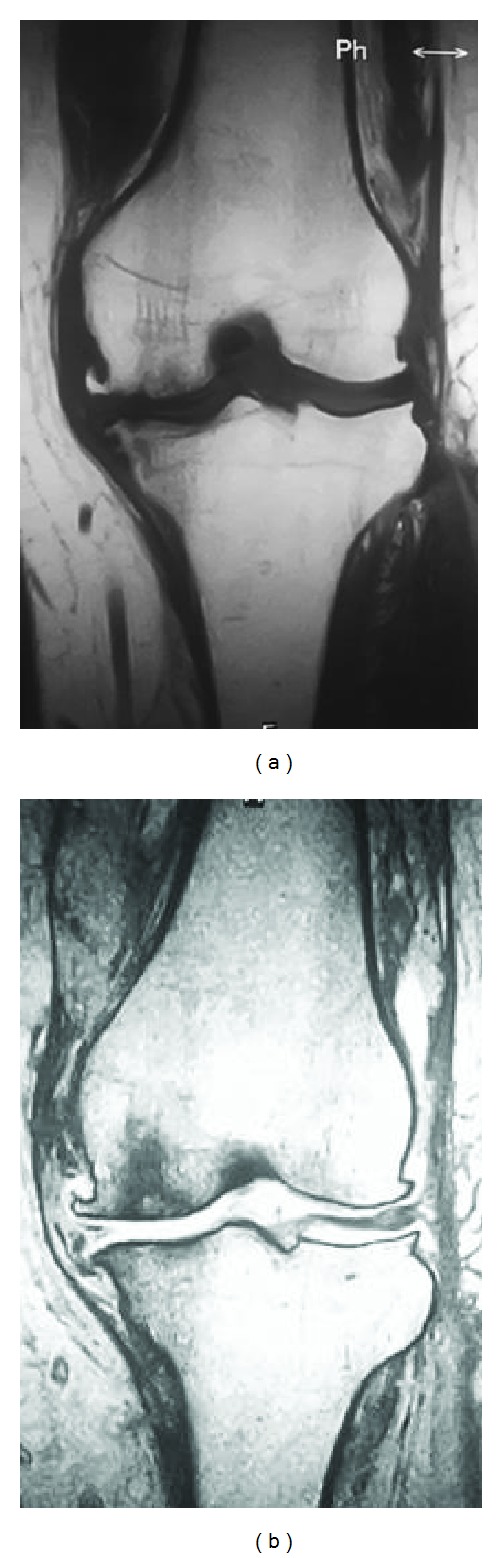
Preoperative magnetic resonance images of the affected knee in Case 2. T1-weighted image shows low signal intensity on the medial femoral condyle and tibial plateau (a). T2-weighted image shows high intensity in the central area of the femoral lesion and the surrounding low-intensity area (b).
